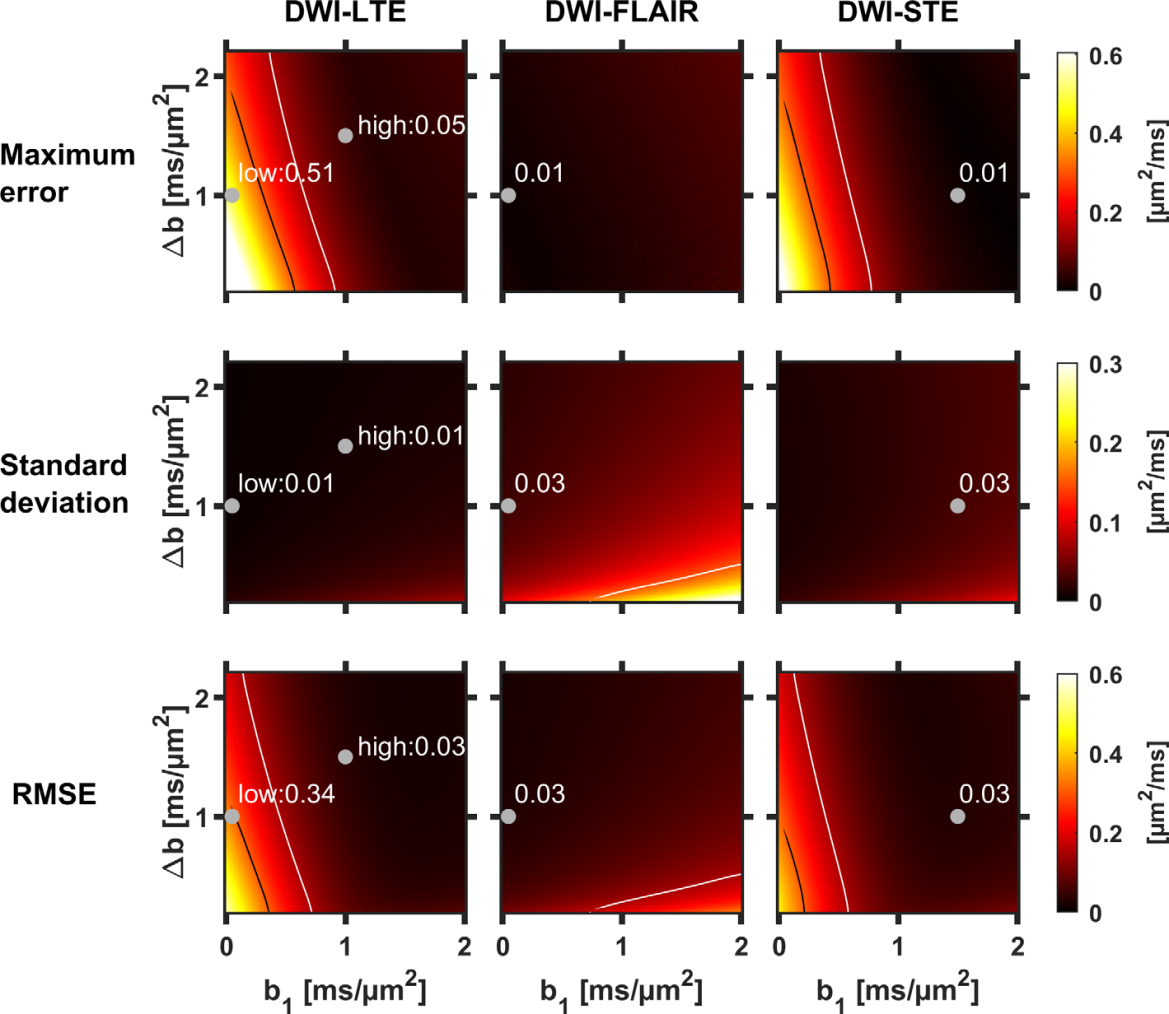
Analysis of accuracy and precision of MD in GM. Maximum errors compared to a noncontaminated signal (top row), SD over noise iterations (middle row), and RMSE compared to noncontaminated signal (bottom row) for DWI‐LTE, DWI‐FLAIR, and DWI‐STE. Points corresponding to the *b*‐value combinations used in vivo are shown in gray, and isolines marking 0.15 μm^2^/ms (white) and 0.3 μm^2^/ms (black) are included. Low *b*‐values yielded large errors for DWI‐LTE and DWI‐STE but not for DWI‐FLAIR. However, SDs were larger for DWI‐FLAIR than for the other techniques. RMSE, root‐mean‐square error.
